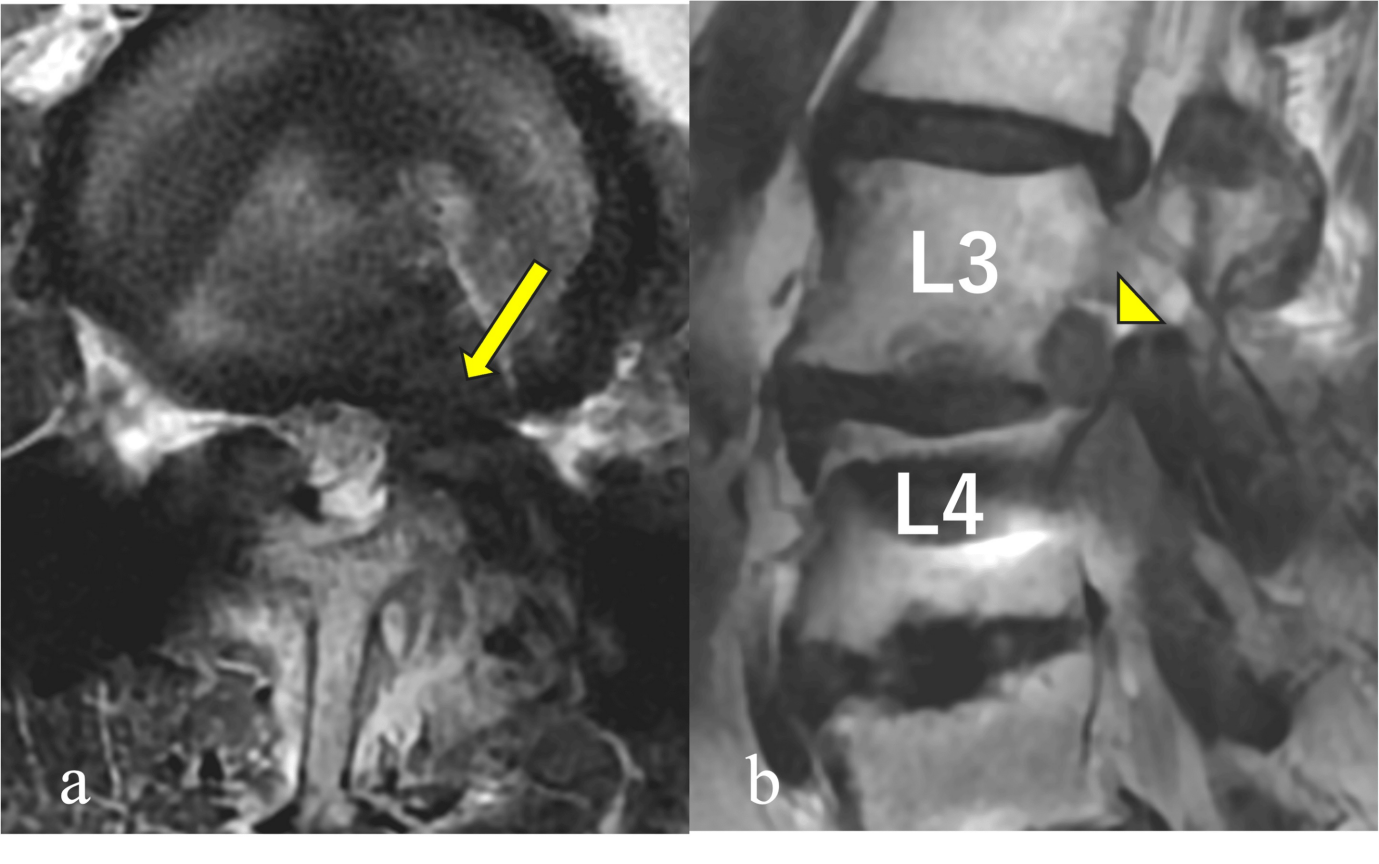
Axial and sagittal MRI findings in case 1 The axial T2-weighted image (a) demonstrates a left L3/4 foraminal disc herniation (arrow), and the sagittal image (b) shows cranial displacement of the L3 nerve root (arrowhead).
